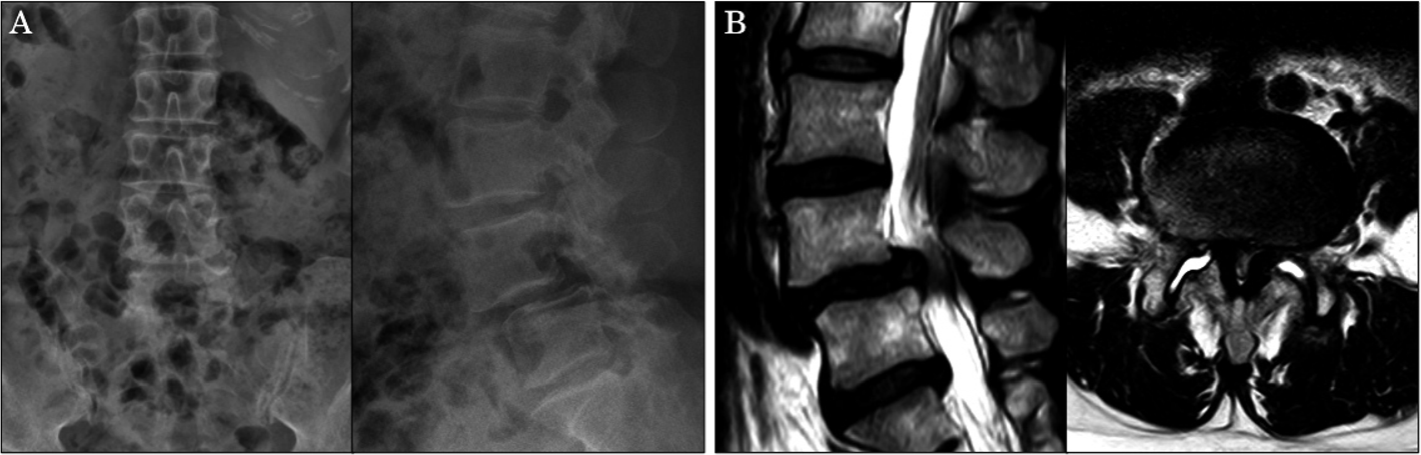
Images at presentation. a Anteroposterior and lateral X-ray films. Note grade II spondylolisthesis at L4/5. b Sagittal and axial magnetic resonance images. The spinal canal is severely compressed at L4/5
